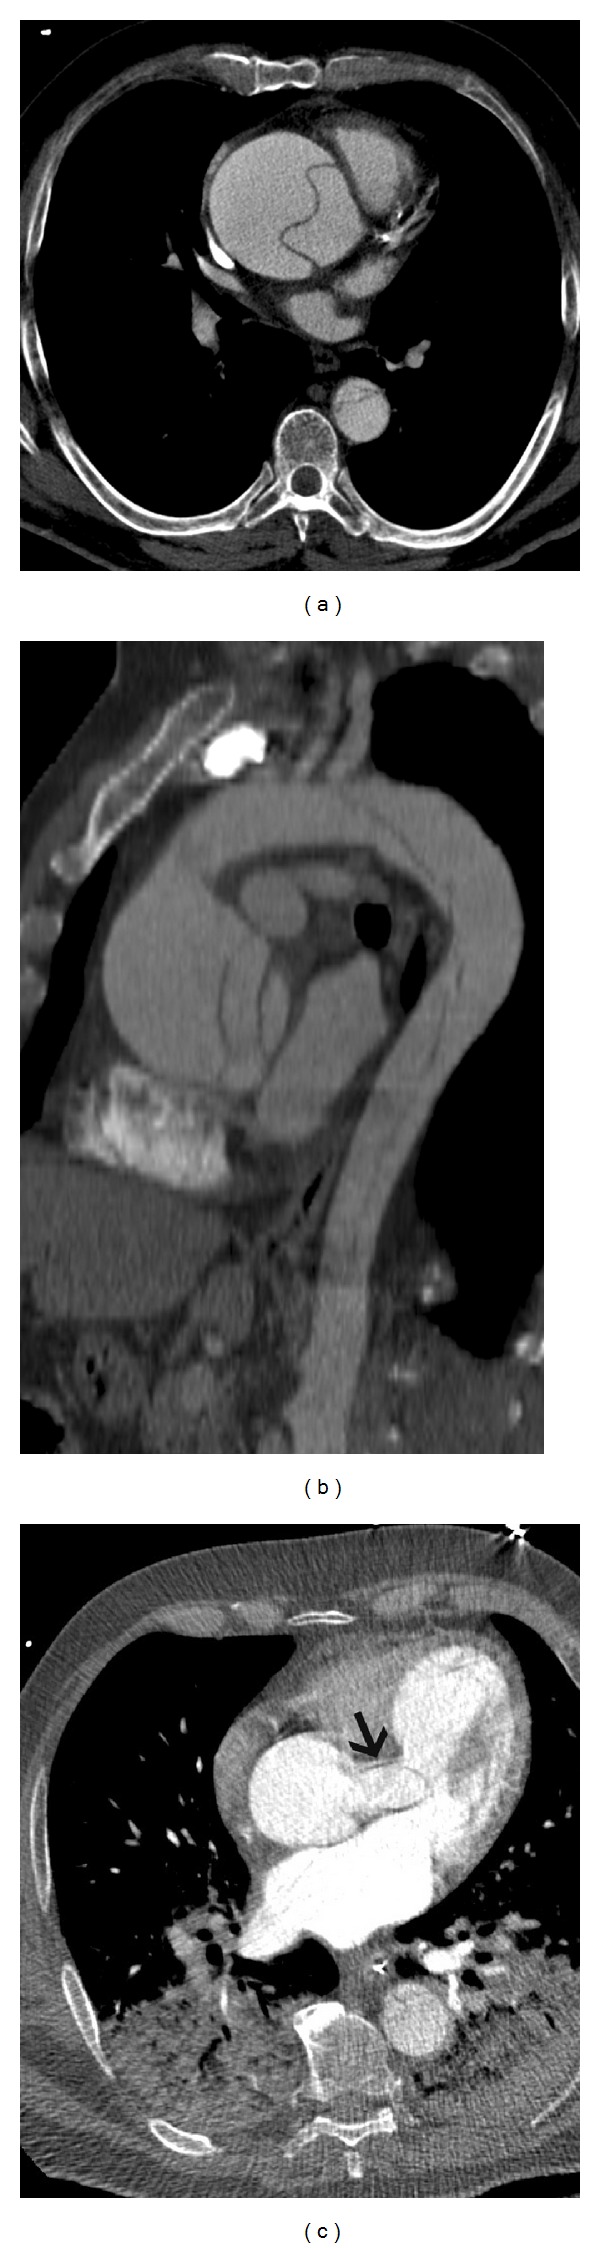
Type  A  dissection—(a) axial postcontrast CT scan in a 56-year-old male shows a dissection flap that involves the ascending aorta and descending thoracic aorta. (b) Sagittal reconstructed post contrast CT image shows the complete dissection flap, beginning from the aortic root and extending across the arch into the descending thoracic aorta. (c) Axial CT scan in another patient shows a Type A dissection flap in the aortic root that is extending into the left coronary artery, which is dilated.
